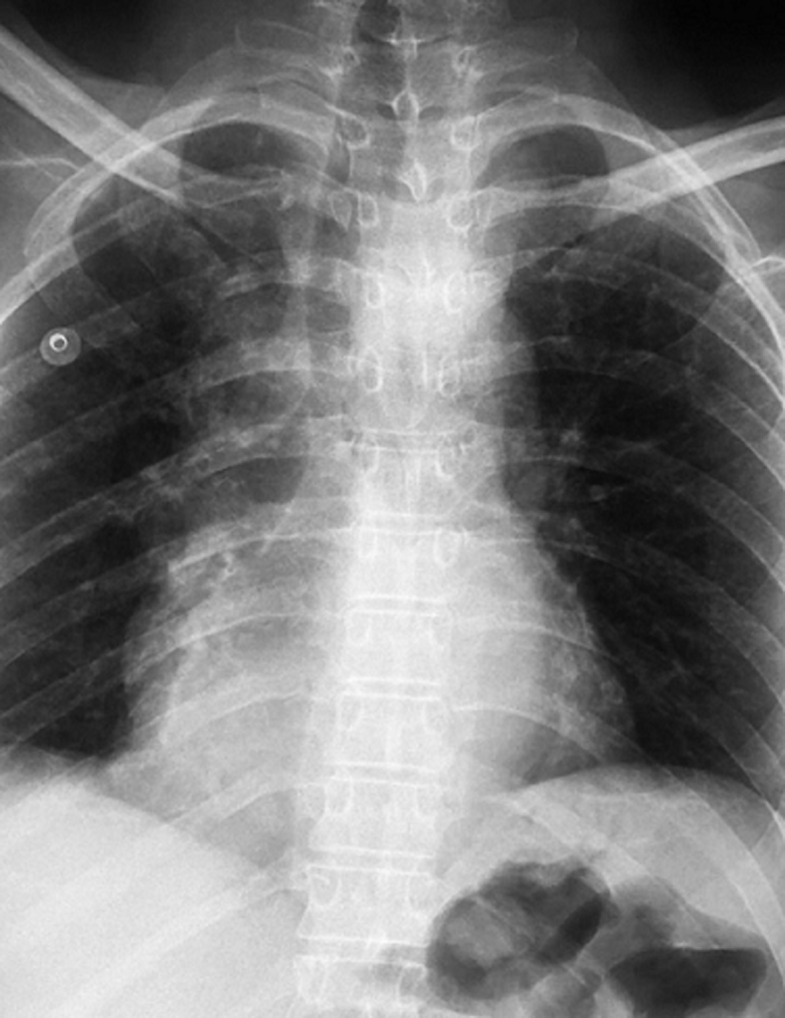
chest X-ray showing homogenous density in the right cardiophrenic angle and trachea deviation
